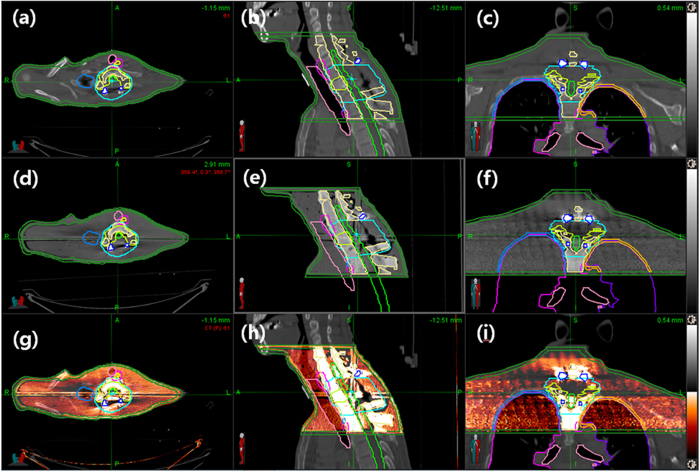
The registered CT of the PSDP_3DP (d–f) was well matched with that of the real patient CT (a–c) in the axial, coronal, and sagittal planes (g–i). The contours of the patient were in good agreement with those of the CT image of the PSDP_3DP (d–f).
